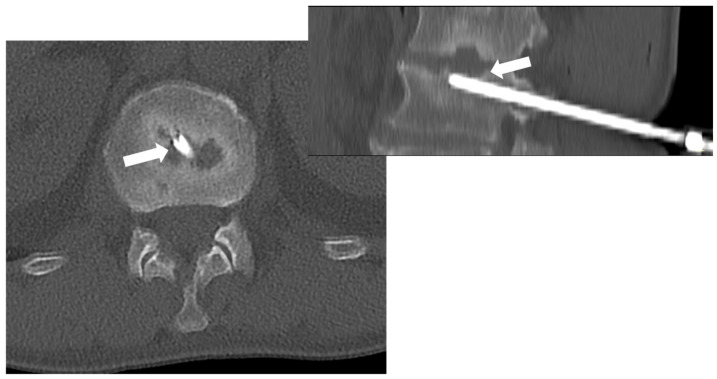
CT-guided biopsy in a 55-year-old male with suspected spondylodiscitis; CT (axial view on the left—sagittal reconstruction on the right) permits to guide the tip of the needle (8 gauge) into the end plate erosion (arrows) adjacent to the disc.
